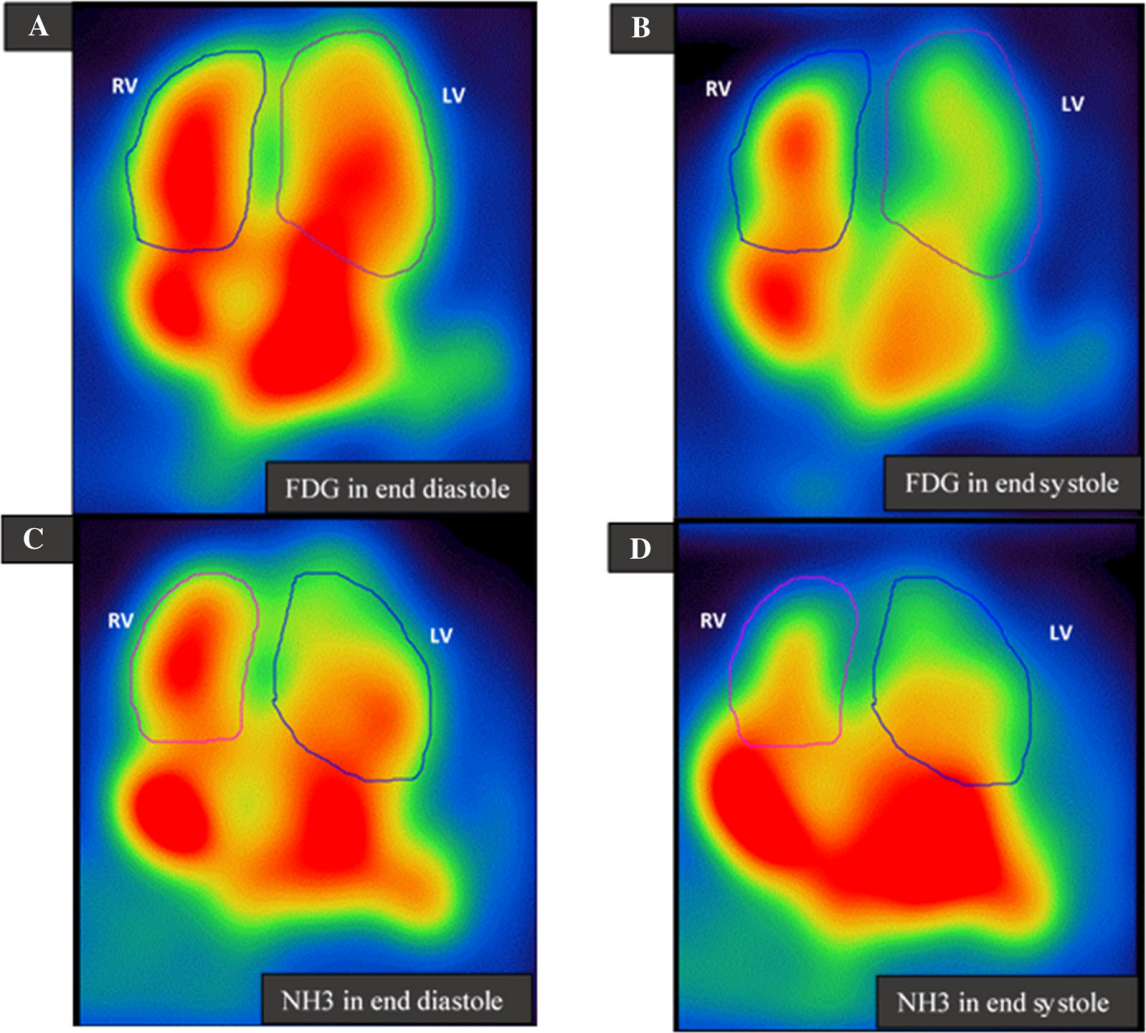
Manual delineation of the right (RV) and left ventricle (LV) from the EDV frames for estimation of first-pass ejection fraction of the left and right ventricle in a 74-year patient with ischemic coronary heart disease. (A) FDG in end-diastolic (ED) and (B) in end-systolic (ES) phase. (C) NH_3_ in ED and (D) in ES
